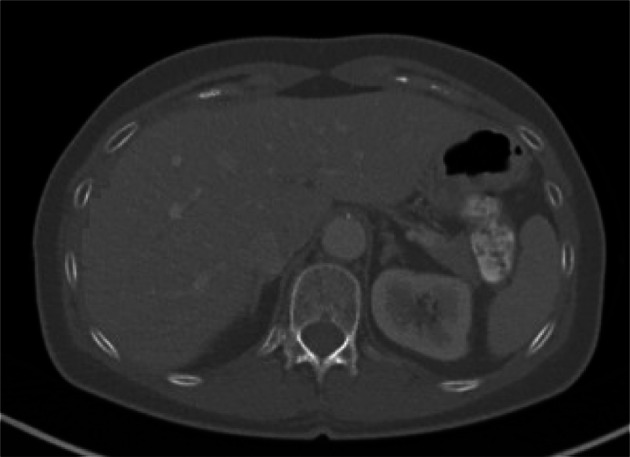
Part of CT image with the graphically removed tumor
